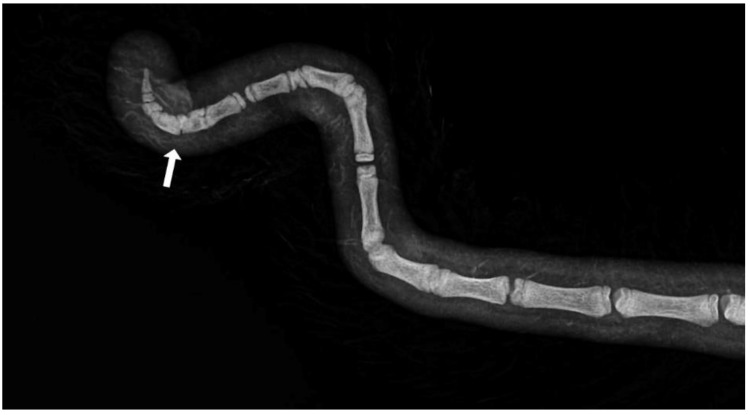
Section of an X-ray of a lamb’s tail with fractured vertebrae.
